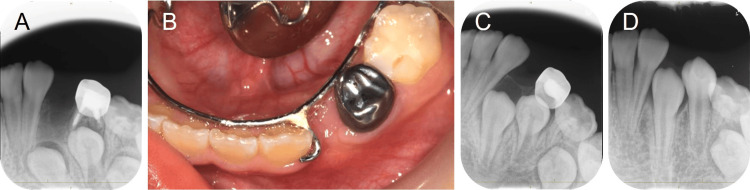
Radiographic examination and intraoral photograph after the extraction of the supernumerary tooth. (A) 8 years and 6 months. (B) 8 years and 7 months. (C) 9 years and 2 months. (D) 9 years and 10 months.
